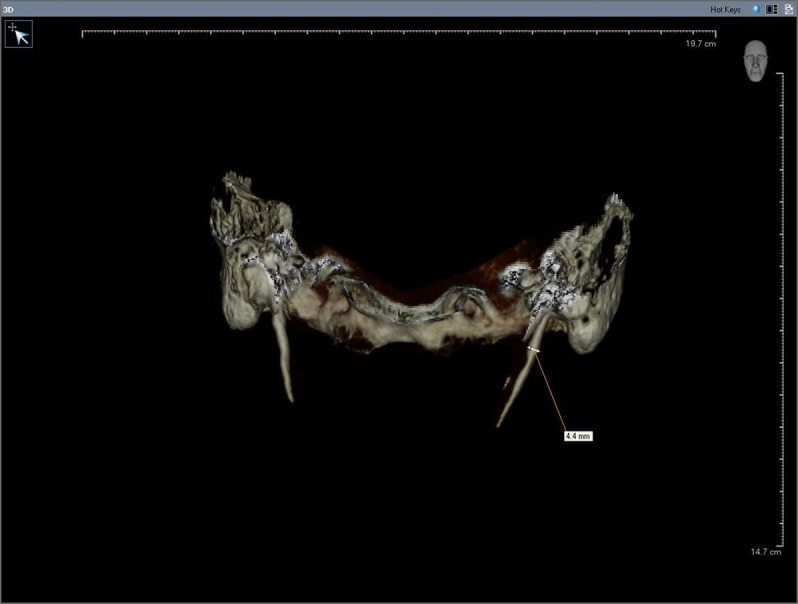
The maximum thickness of SHC ossification on CBCT
